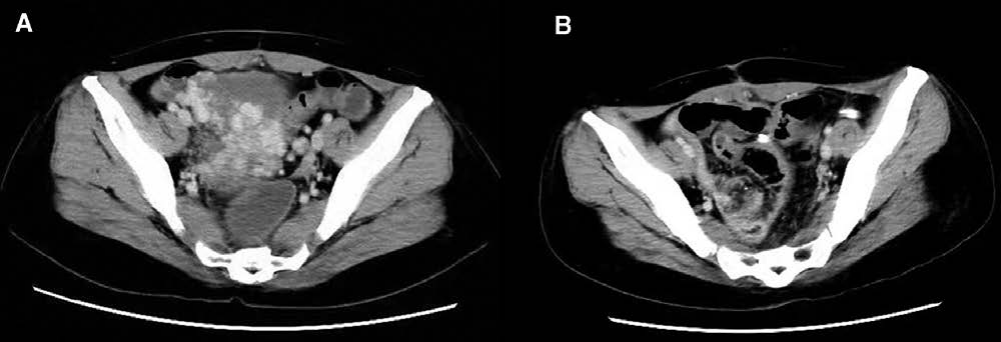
Computed tomography: (A) multiple tortuous and dilated vascular shadows in the pelvic cavity; and (B) no residual varices were observed after sigmoid colon resection.
